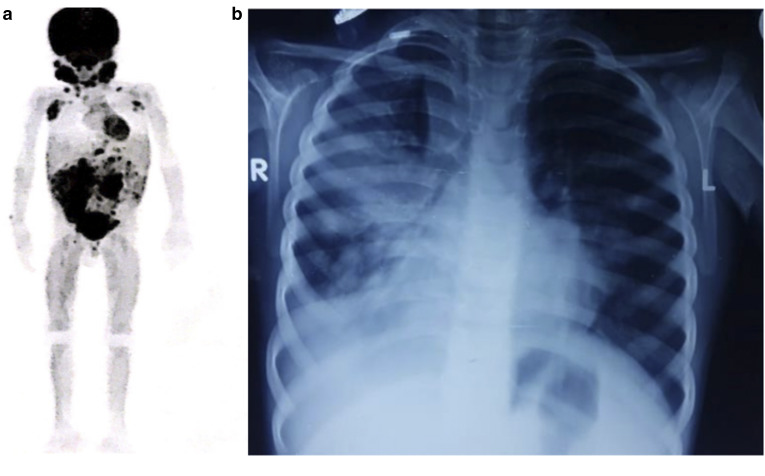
(a) Fluorodeoxyglucose positron emission tomography of P4 showed multiple enhancing enlarged discrete conglomerated supra- and infradiaphragmatic lymph nodes, bulky adenoids, bilateral tonsils, and ground glass nodules in bilateral lung parenchyma. (b) Chest radiograph of P3 showed right lobar pneumonia.
